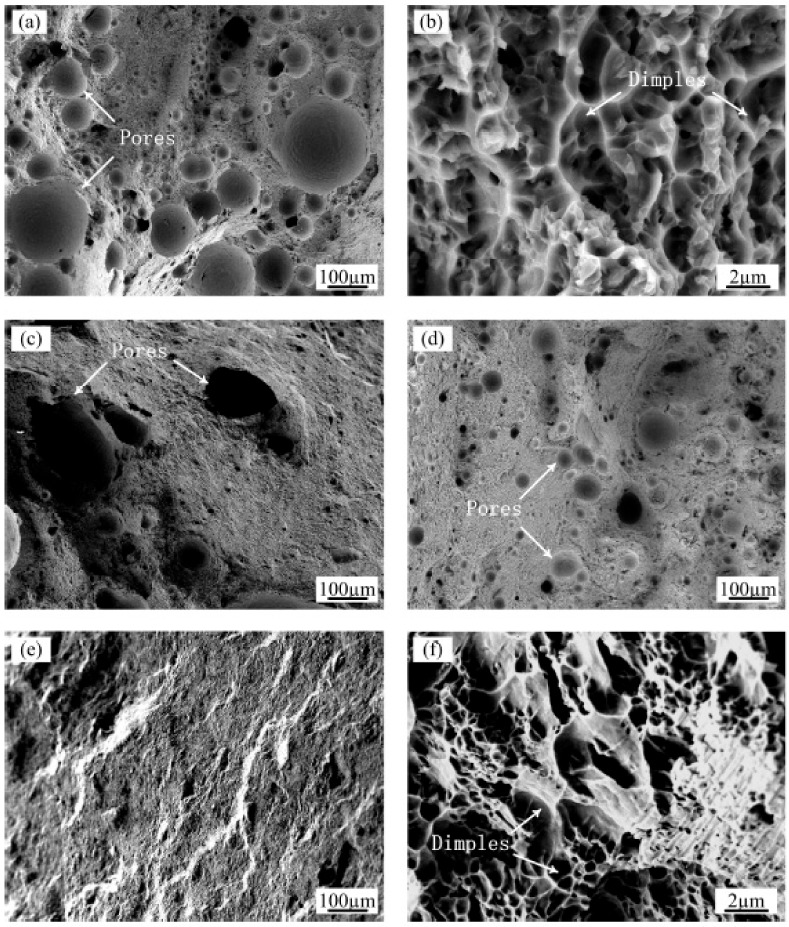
SEM images of the tensile fracture surfaces of (a) The AS sample at low magnification and (b) high magnification; (c) The AS sample with ultrasonic action at low magnification; (d) The RM sample at low magnification; (e) The RM sample with ultrasonic action at low magnification and (f) high magnification.
